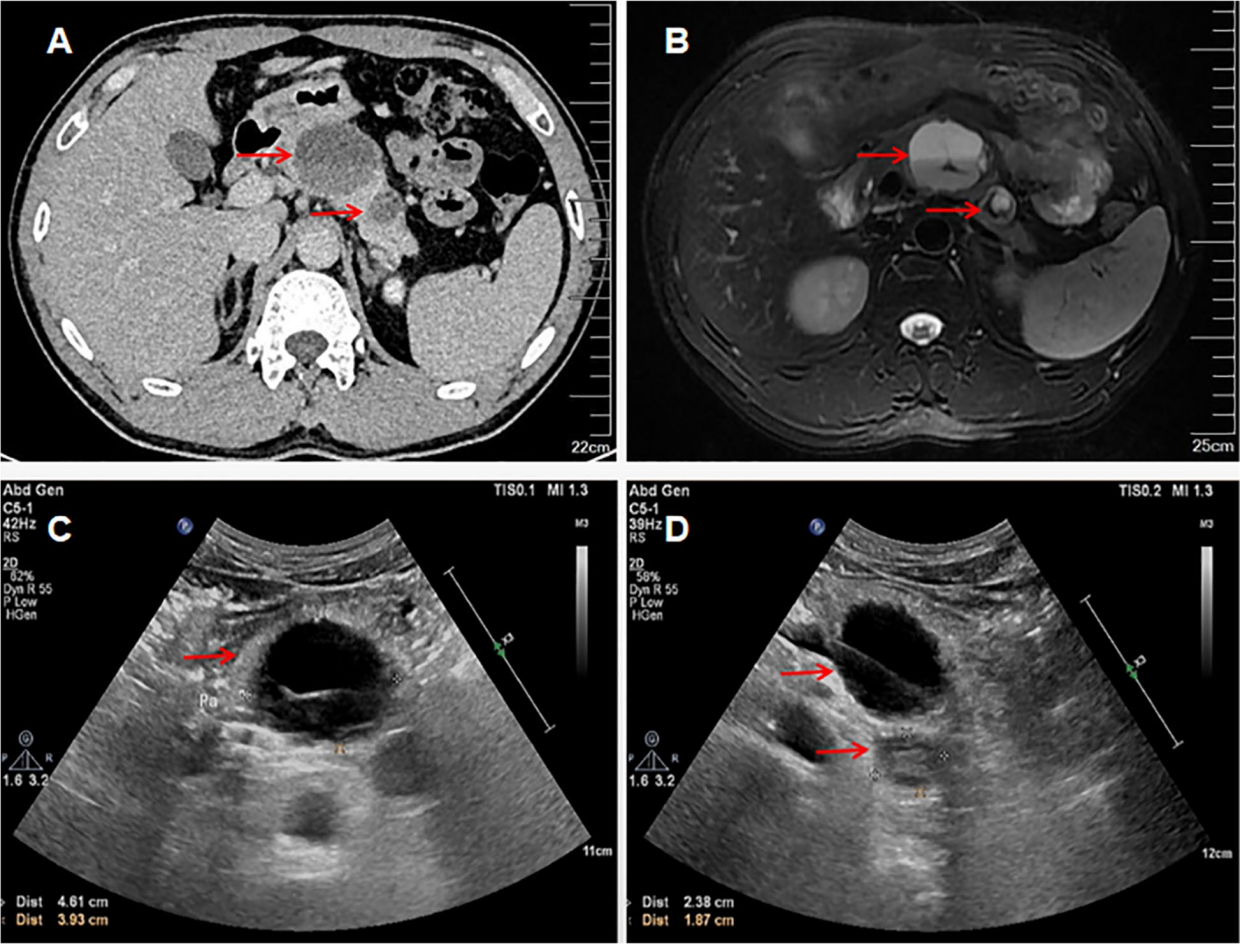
Image of pleomorphic sarcoma of the pancreas. A The abdominal CT scan showed two hypointense lesions in the pancreas body and tail. B MRI revealed two round cystic abnormal signal involving the body and tail of the pancreas. C, D Ultrasound imaging exhibited a cystic echo in the body of the pancreas with separation and hypoecho in the tail. (the red arrow indicates the masses)
